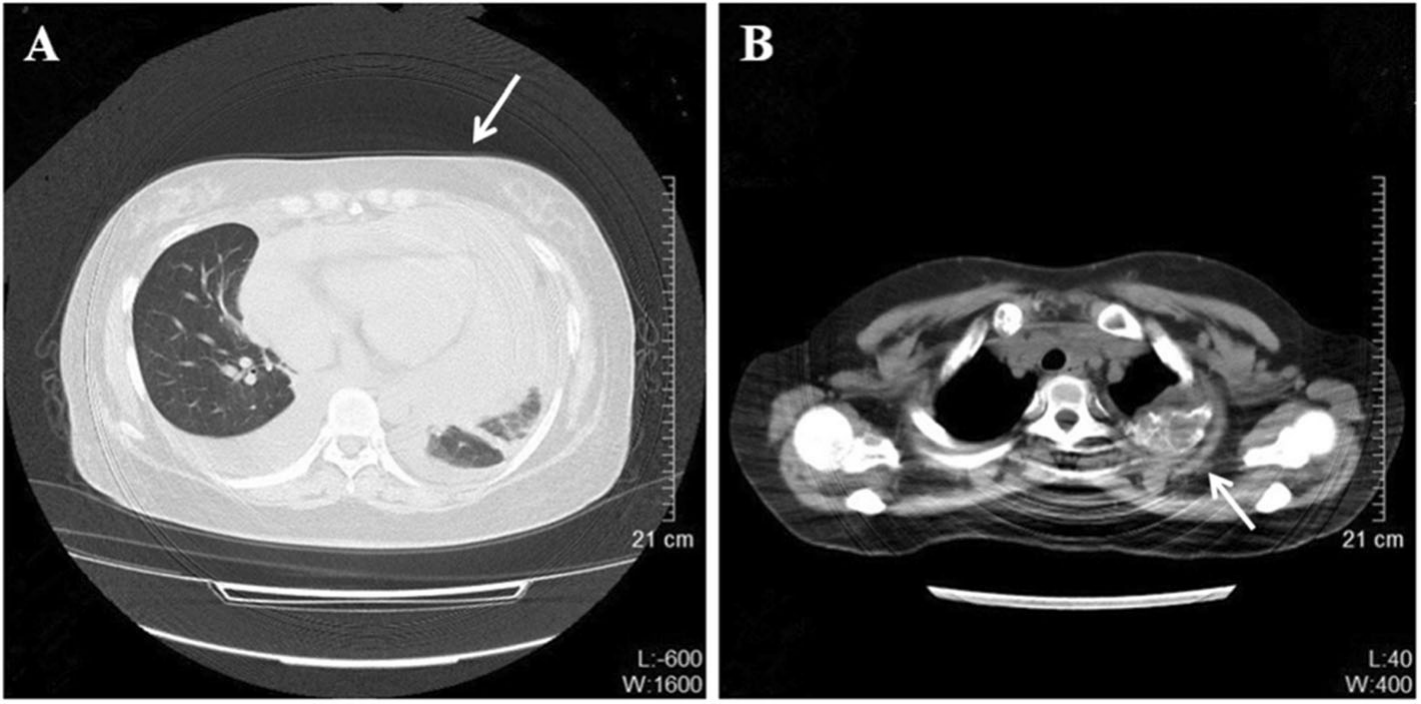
Chest computed tomography obtained at time of initial presentation showing massive pericardia and pleural fluid (white arrow) (a) in addition to pleural effusion and an osteolytic lesion on the second left rib (white arrow) (b)
